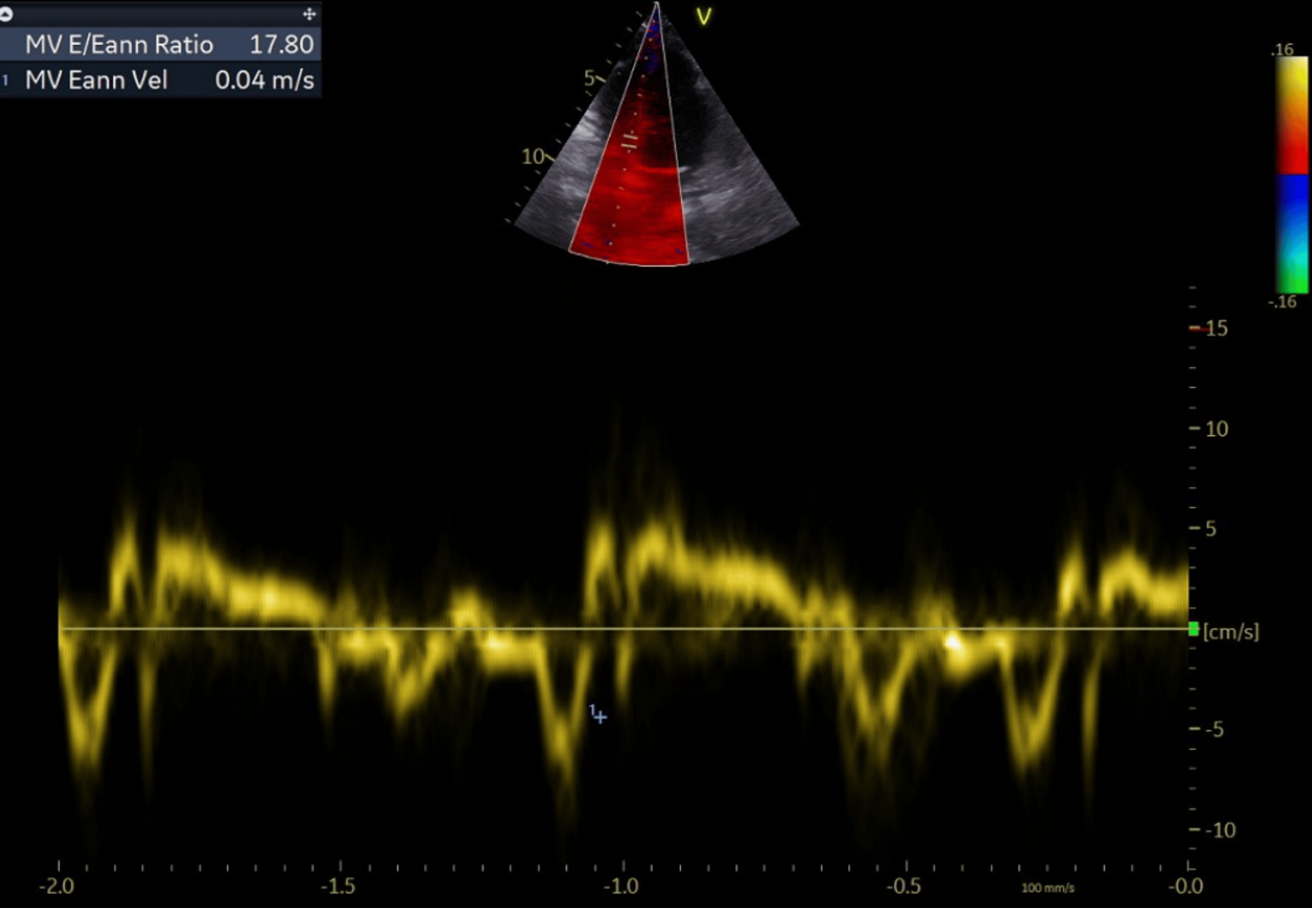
Evaluation of diastolic dysfunction (E/E’=17) The E wave is the early diastolic filling wave seen on Doppler interrogation of the mitral valve. The E' wave is acquired in tissue Doppler either of the basal or the lateral wall (in our case we used the mean value) of the mitral annulus. Values greater than 15 suggest that left ventricle end-diastolic pressure is elevated.
